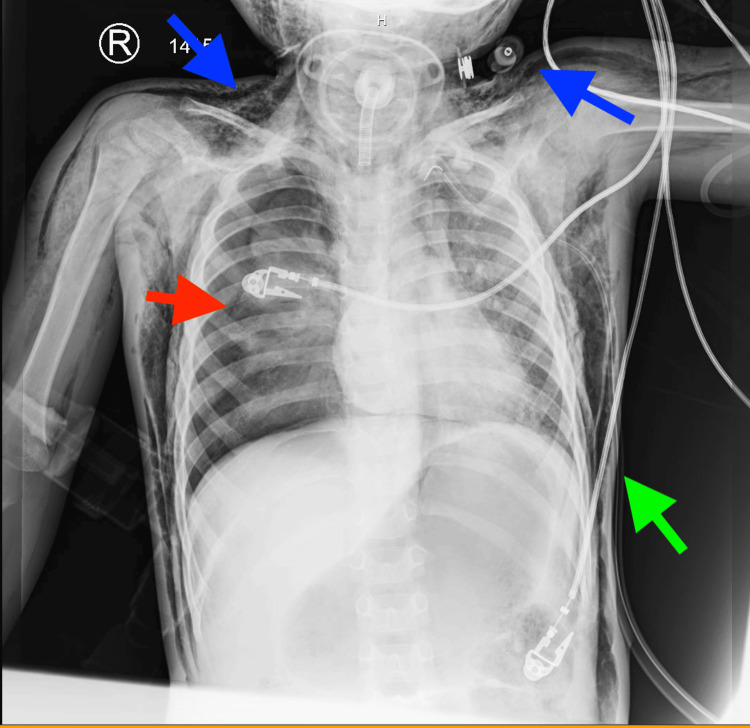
Follow-up chest radiograph findings Demonstrating re-expansion of the left lung with a left-sided chest tube in place (green arrow) and interval development of a right-sided pneumothorax (red arrow). Persistent subcutaneous emphysema is again noted (blue arrows).
